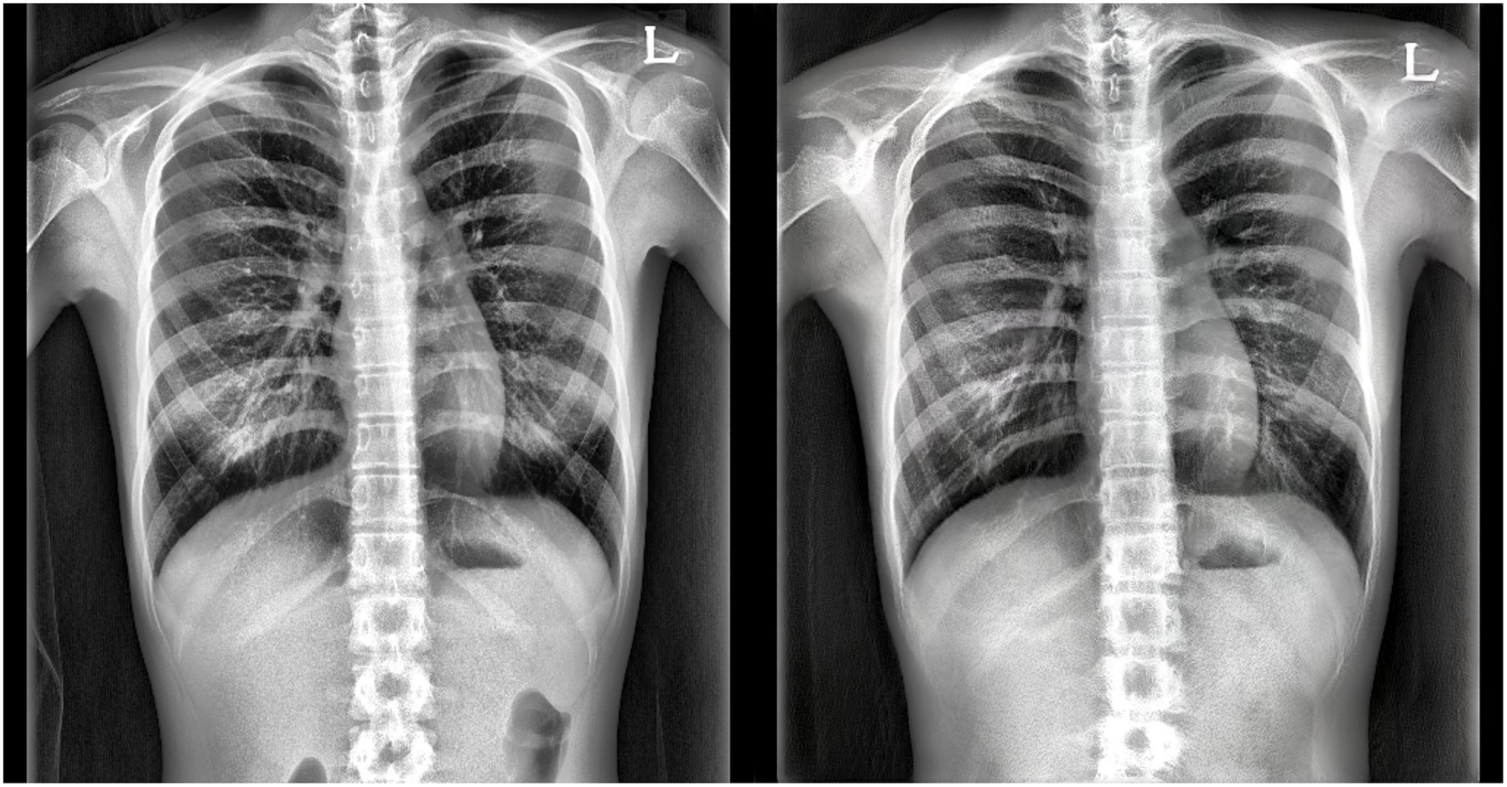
Example of a well-reconstructed normal sample. (Left) original chest X-ray. (Right) Reconstructed image using the GAN inversion technique. The encoding generator was only trained with chest X-ray images with scoliosis.
